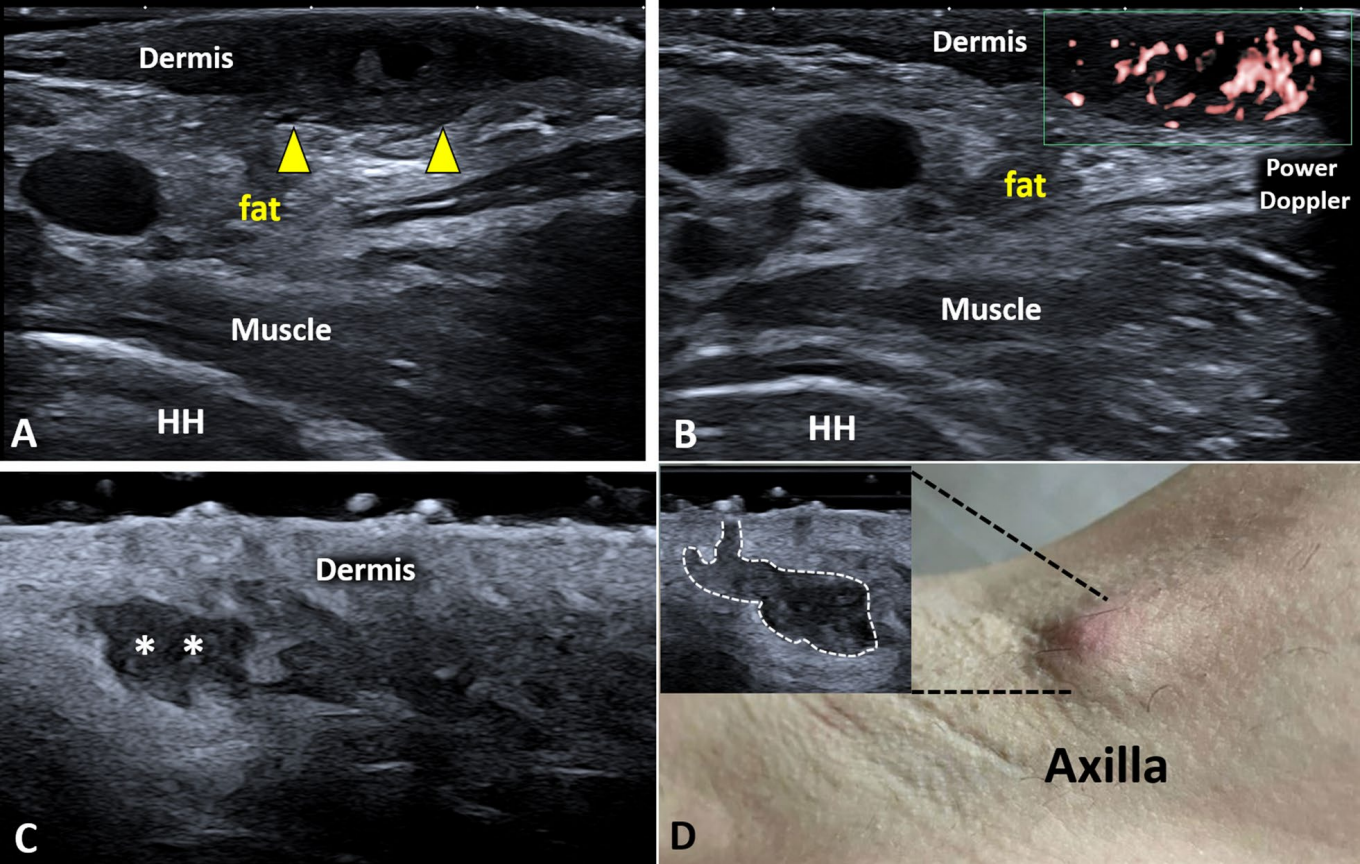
Hidradenitis suppurativa of the axilla. Massive hypoechoic thickening *(yellow arrowheads)* of the dermis (A) with pathological hypervascularization on high-sensitivity color Doppler (B) can be promptly observed at the level of the axillary skin. Very high-frequency ultrasound probes (C, D) better define additional sonographic signs of hidradenitis suppurativa as the superficial, saclike fluid collections *(white asterisks)* and the ballooning of hair follicles *(white dotted line)*. *HH* Humeral head
